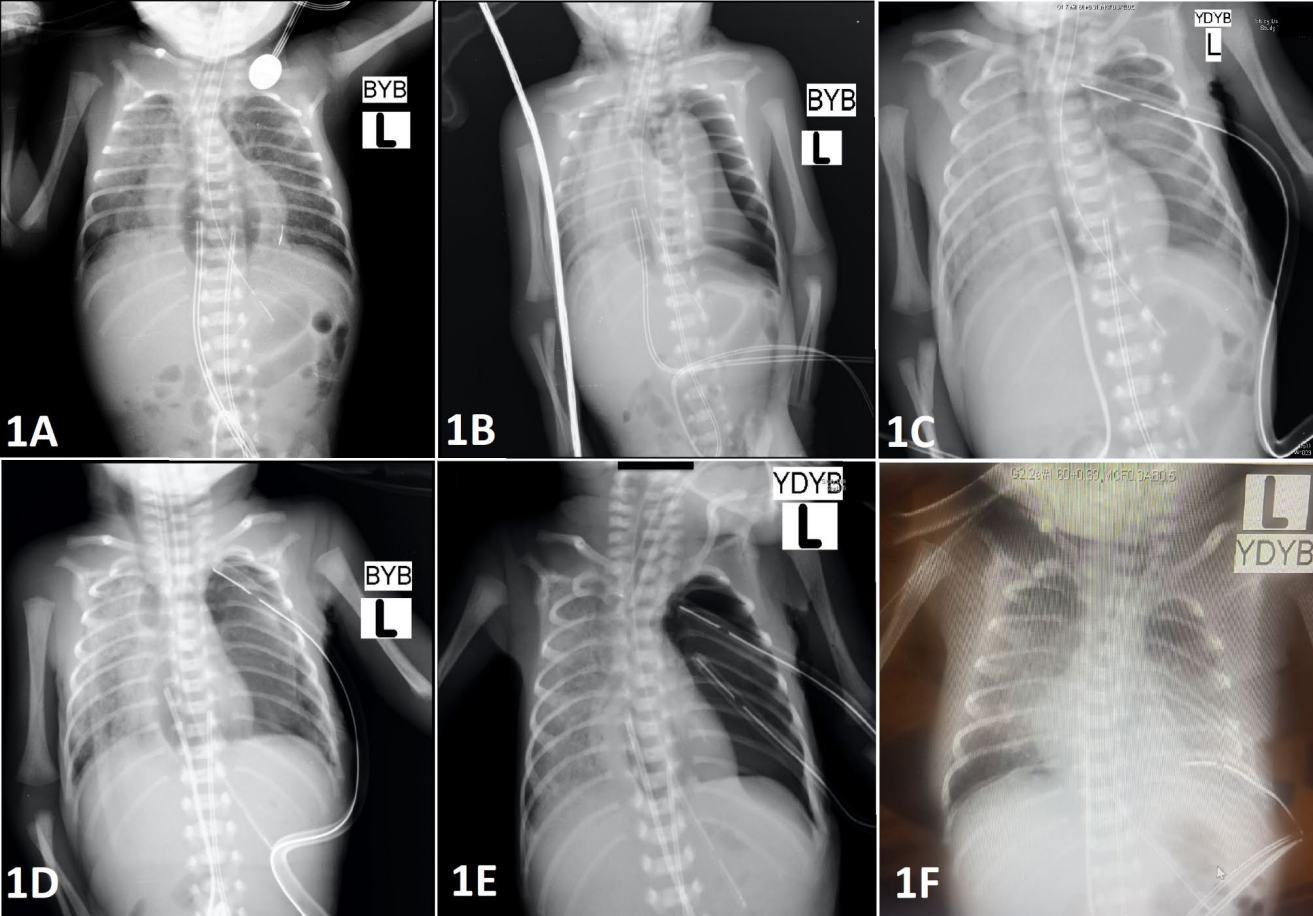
A: Initial X-ray showing pneumomediastinum. B: Left pneumothorax and mediastinal shift to the right side. C: Expanded left lung after thorax tube placement. D: Repeat pneumothorax in the left lung. E: Repeat pneumothorax, post-second thorax tube placement. F: After 1st thoracotomy, fully expanded left lung
